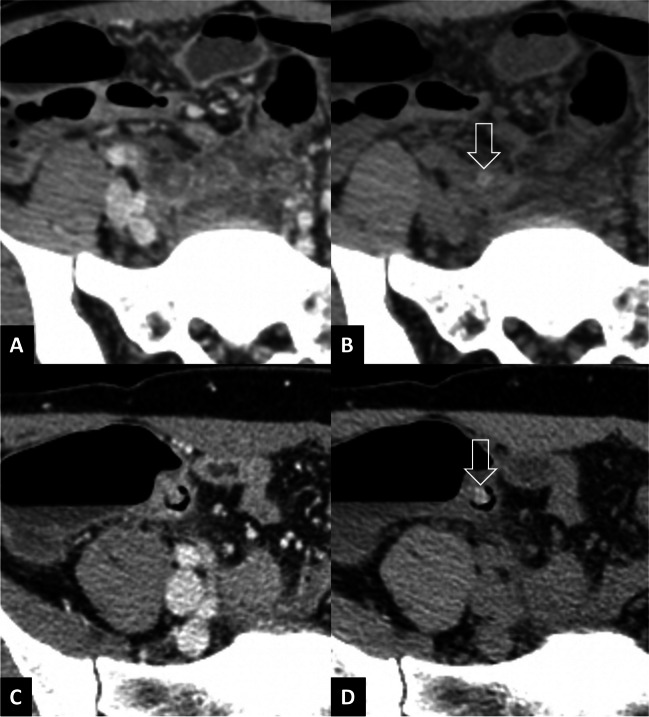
Example of two cases with appendicoliths (arrows) undetected on portovenous-phase CT (A, C) but visible on noncontrast CT (B, D)
